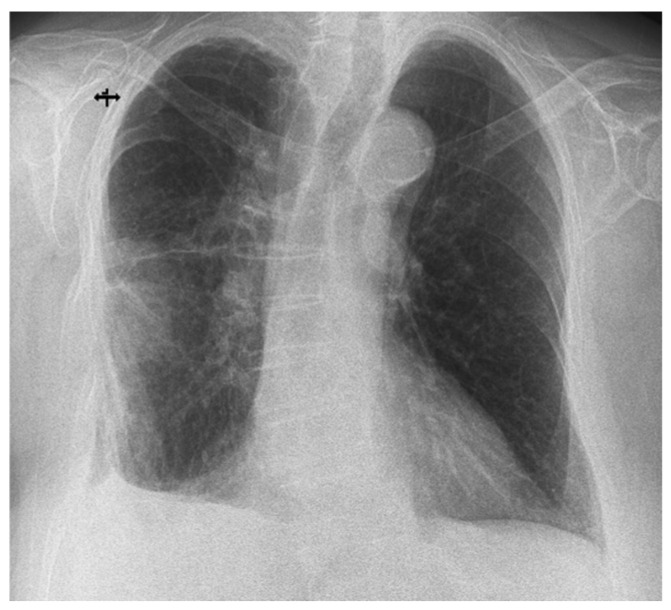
Postoperative chest radiography demonstrated satisfactory re-expansion of the right lung, with no evidence of pleural effusion. Mild residual interstitial opacities were observed in the right lower hemithorax, most likely representing postoperative sequelae and/or subsegmental atelectatic changes Source: authors’ archive.
